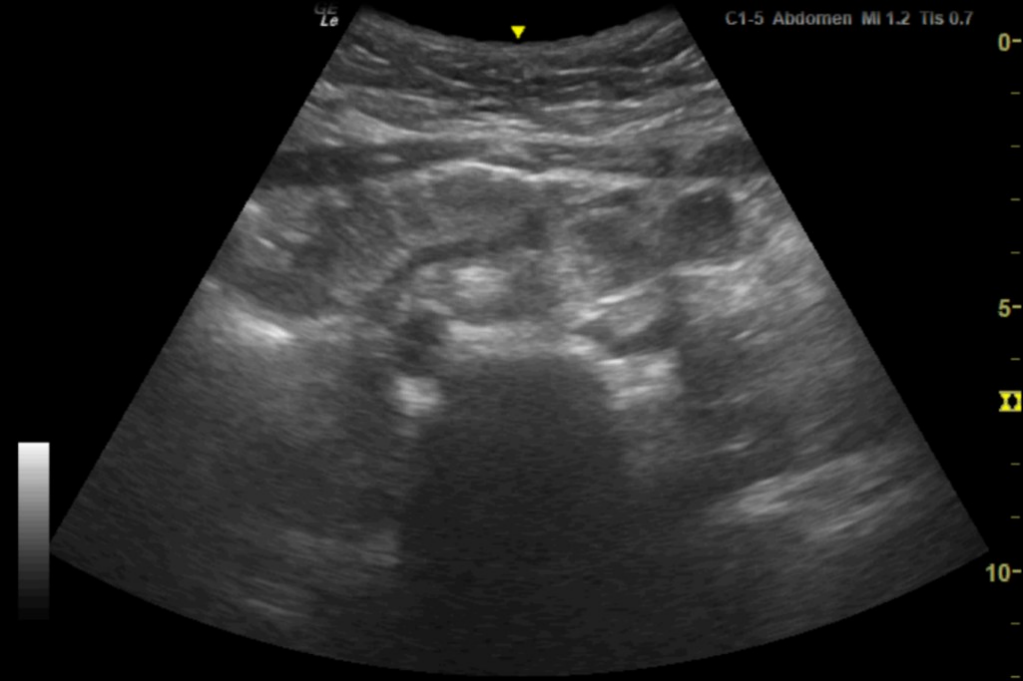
Point of care ultrasound (POCUS) image of lower abdomen in transverse orientation using the 1-5 MHz curvilinear probe. There is a suspicion of a small amount of free fluid in the right lower quadrant.
